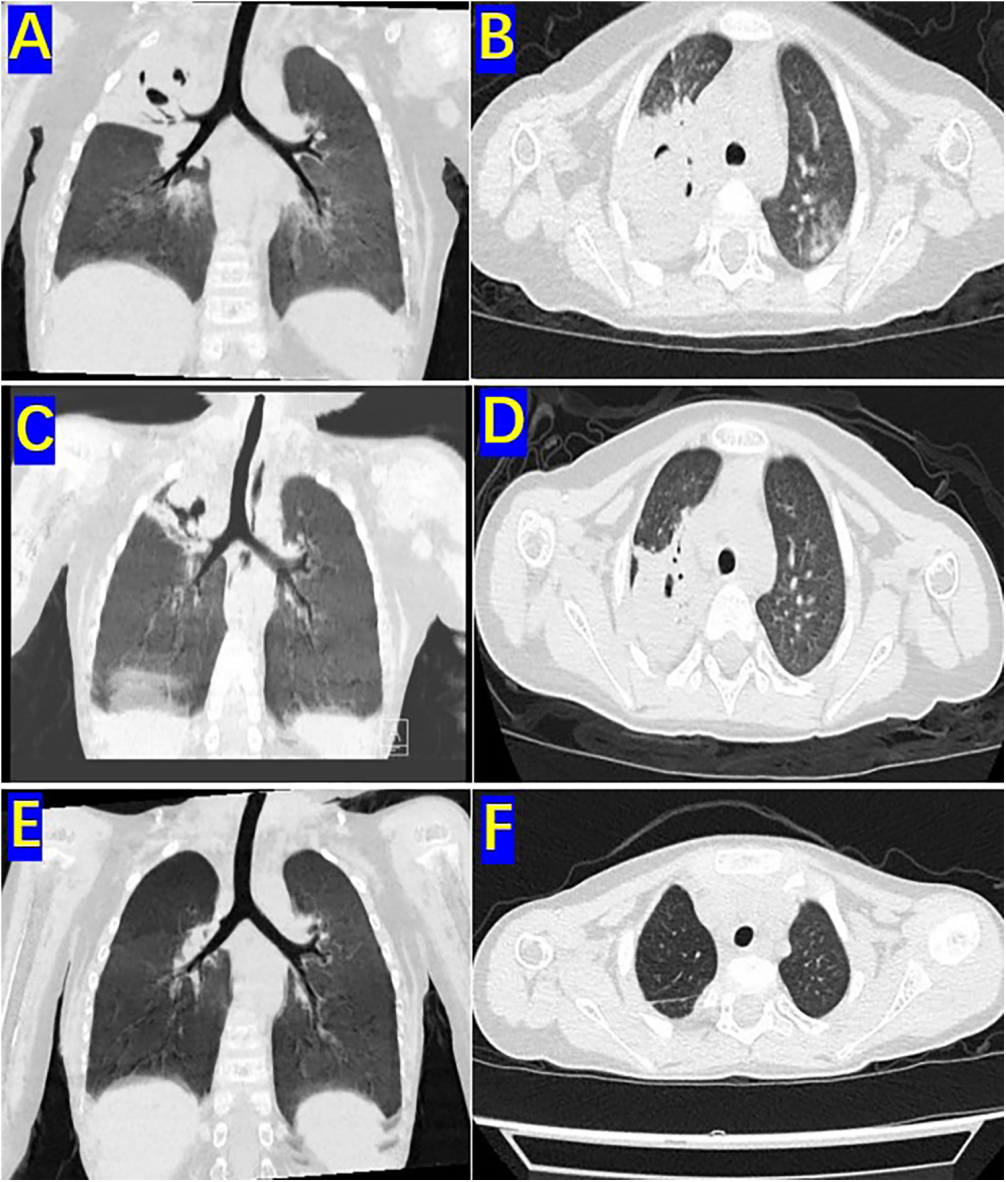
Pre-bronchoscopy treatment CT, preoperative CT and One-month postoperative CT: (A) sagittal view of the high-resolution chest CT lung window before two bronchoscopic bronchoalveolar lavages. (B) Coronal view of the right upper lobe on high-resolution chest CT lung window before two bronchoscopic bronchoalveolar lavages. (C) Sagittal view of the high-resolution chest CT lung window before surgery. (D) Coronal view of the upper lobe in the high-resolution chest CT lung window before surgery. (E) Sagittal view of the high-resolution chest CT lung window 1 month after surgery. (F) Coronal view of the upper lobe in the high-resolution chest CT lung window 1 month after surgery.
